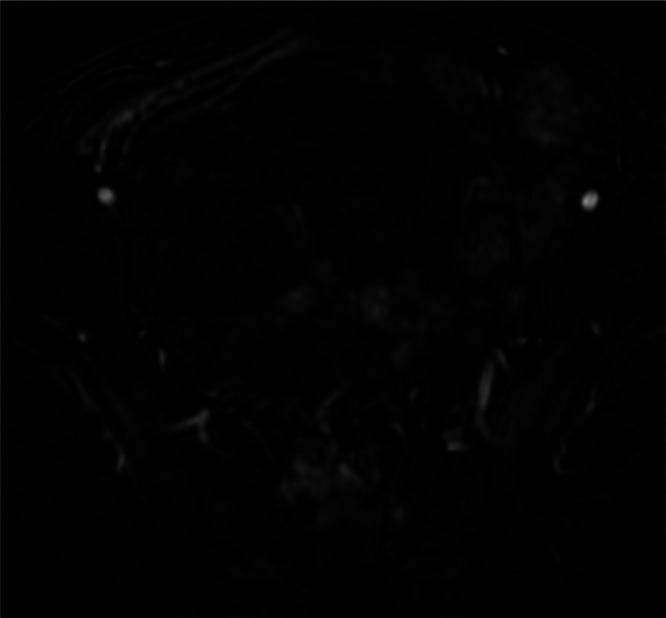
Subtracted image from a 10-month follow-up contrast-enhanced pelvic MRI at the level of the uterine arteries demonstrated the hypovascular nature of the AAM, which did not significantly reduce in size despite leuprolide therapy.
